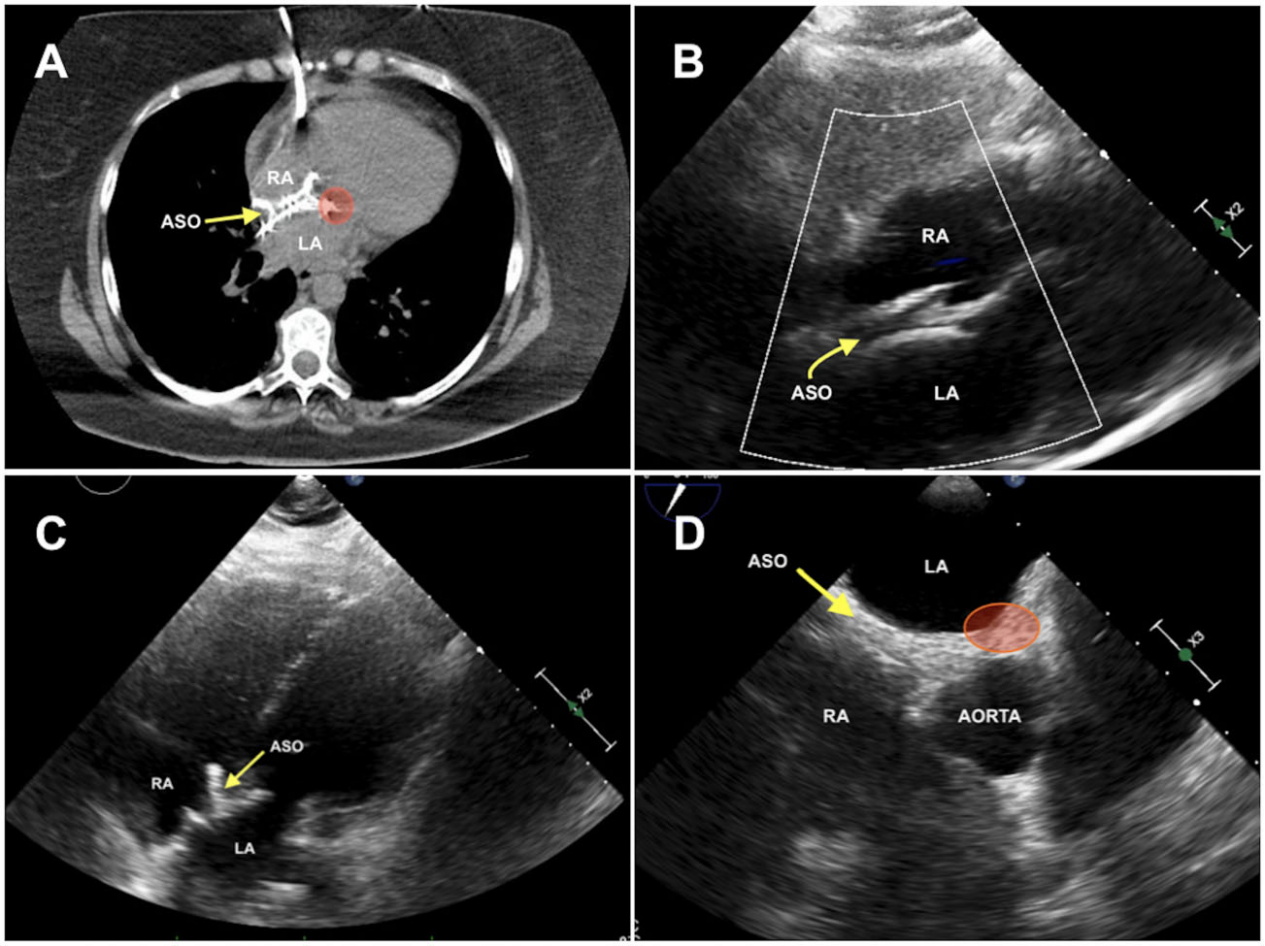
Imaging investigations. The area of injury is demonstrated with a red circle. (A) Chest CT scan. (B) TTE-subcostal four-chamber view. (C) TTE-apical four-chamber view. (D) TOE-aortic valve short axis. ASO: Amplatzer Septal Occluder; CT: computed tomography; LA: left atrium; RA: right atrium; TOE: transoesophageal echocardiogram; TTE: transthoracic echocardiogram.
